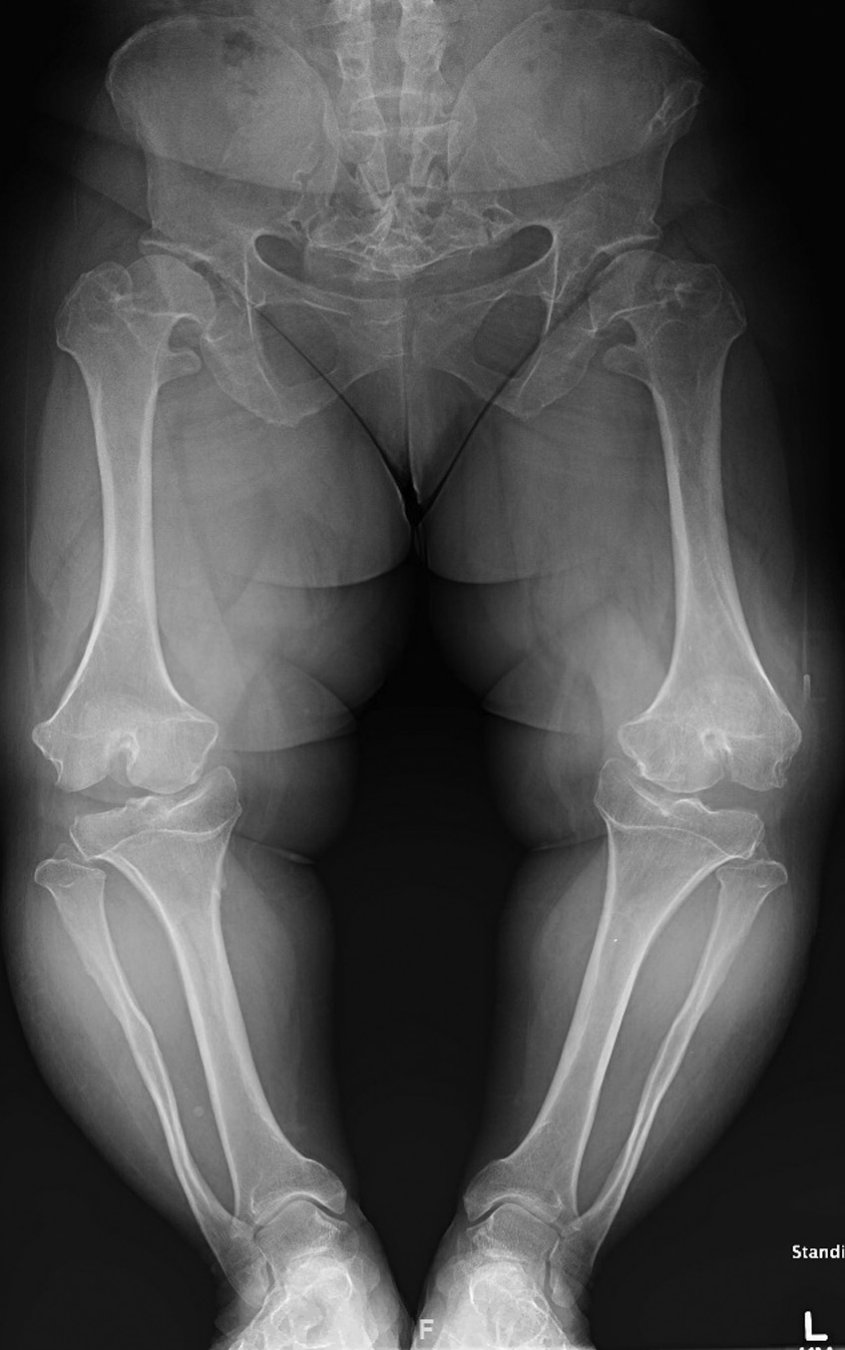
AP bone length radiograph. Characteristic rhizomelic shortening and metaphyseal changes associated with achondroplasia. Notable medial joint space narrowing with lateral widening suggestive of instability.
